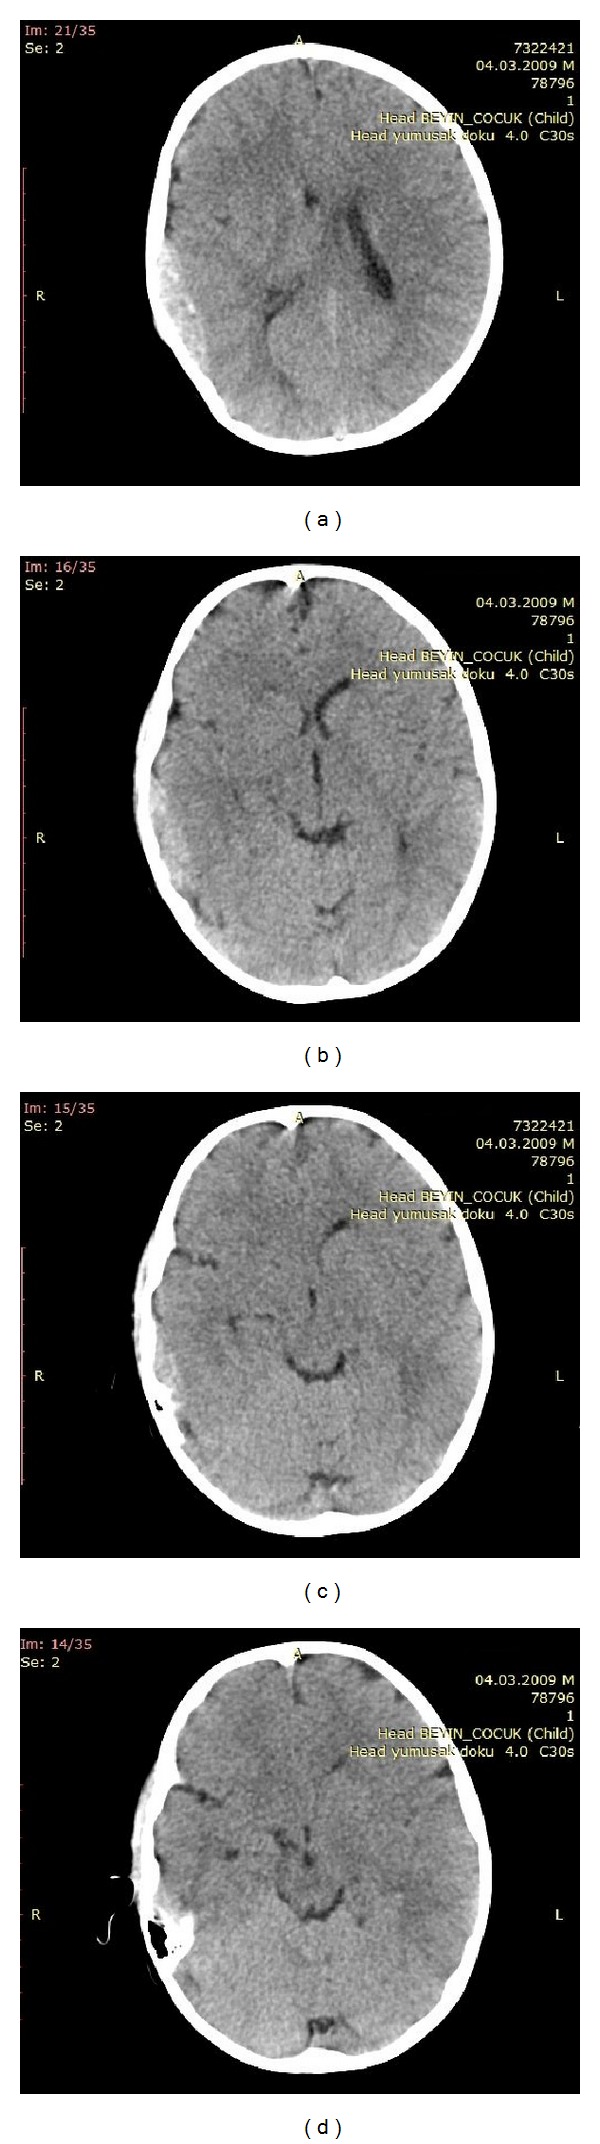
Axial CT image showing right sided epidural hematoma. (a) Inferior border of the hematoma at level of mastoid air cells. (b) Hematoma continuity at right temporal region. (c) Level of lateral ventricles. (d) Level of centrum semiovale.
